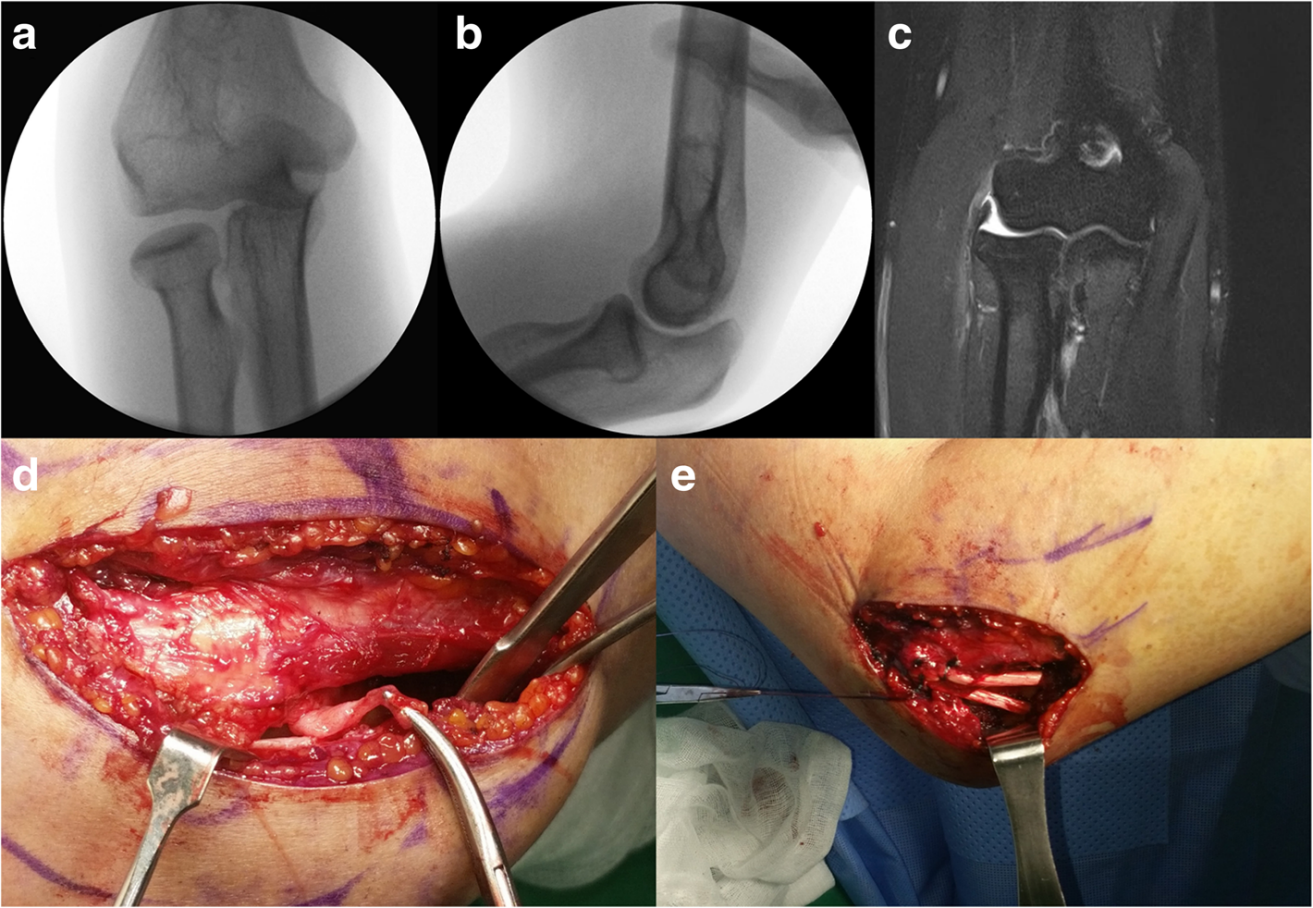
a The 2.1 mm widening of the radiocapitellar joint was identified in the varus stress view of a 38-year-old man (patient #5). b The radial head was shifted posteriorly in the posterolateral pivot shift test. c T2-weighted coronal magnetic resonance image showed rupture of the lateral collateral ligament. d Intraoperatively, the definite lateral and lateral ulnar collateral ligaments were not found, except for thin fibrous tissue. e The lateral ulnar collateral ligament was reconstructed with a tibialis anterior allograft
